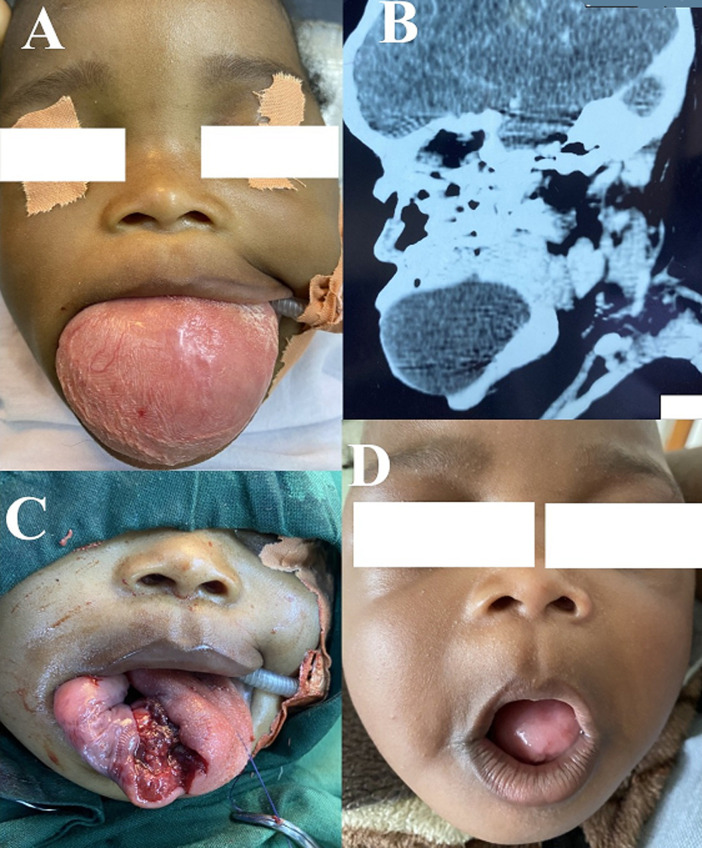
A) pre-operative aspect of mass; B) sagittal computed tomography (CT) scan showing intra-lingual cystic lesion; C) intra-operative view after excision; D) three weeks post-operative
